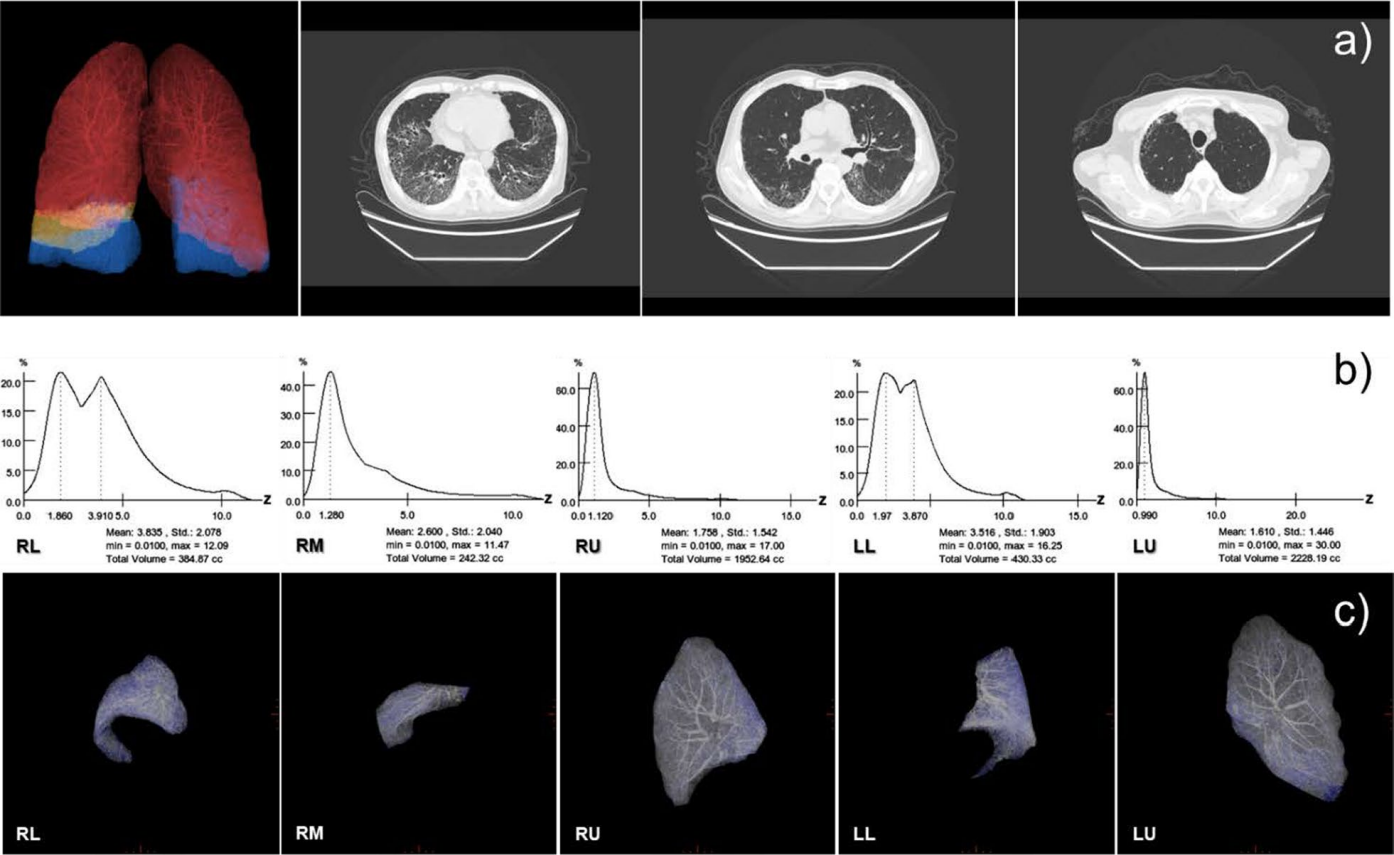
The component distribution histograms of DECT parameter in a 66-year-old man, diagnosed with interstitial lung disease associated with MCTD (PM/DM and Sjögren syndrome). Row a presents colored split lung lobes in 3D and axial computed tomographic images of the chest at the three levels. The histograms of Z_eff_ value were shown in Row b. Compared with each other, shape of the histogram in lower lung lobes with complex lesions was wider, the mean value was higher and the wave peaks are more than those of the middle and upper lung lobes. In RL, RM, RU, LL, LU order, the mean Z_eff_ value was 3.835, 2.600, 1.758, 3.516 and 1.610 respectively. Row c presented each lung lobe along with bronchovascular bundle in three-dimension (3D), and blue represents the interstitial lung abnormalities
